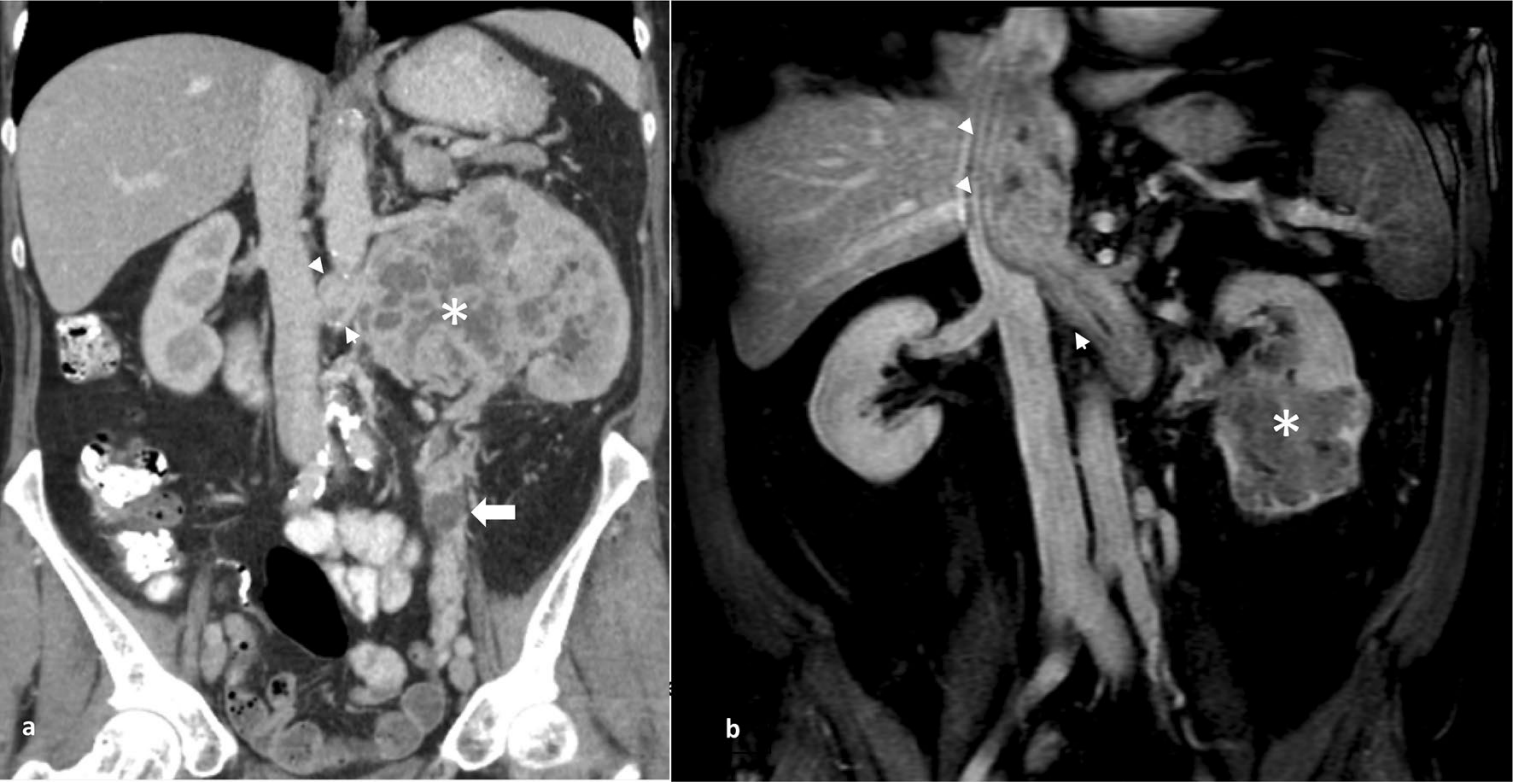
Coronal portal venous phase CT depicting a large left lower pole renal tumour (star) with direct extension into the renal vein (arrowheads) and along the gonadal vein (filled arrow). Coronal fat-saturated T1 weighted MRI images following intravenous gadolinium in a different case demonstrate enhancing tumour thrombus within the left renal vein and extending into the infradiaphragmatic vena cava (stage T3b)
